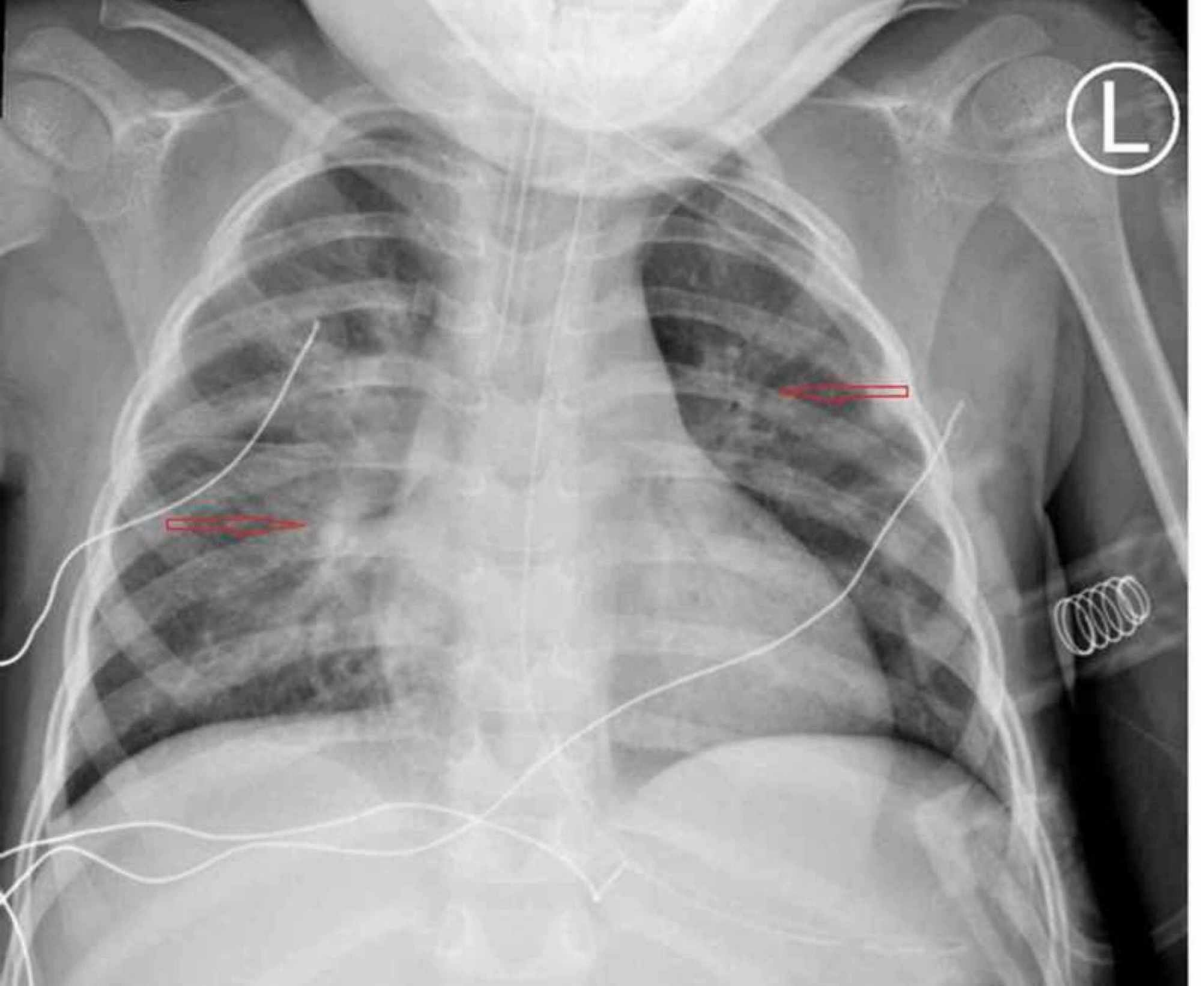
Chest X-ray showing bilateral patchy atelactasis
